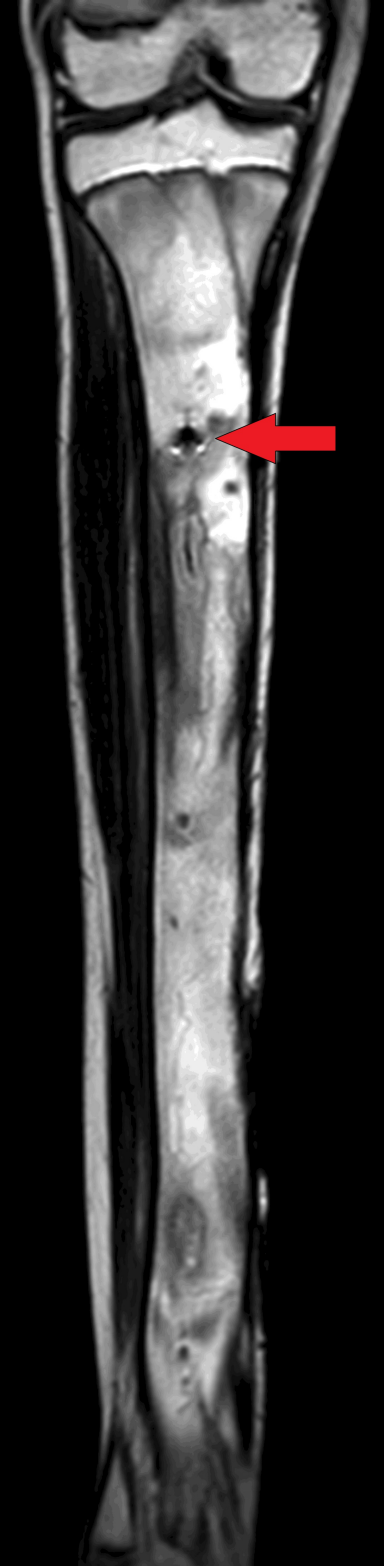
Coronal T1WI MRI of the tibia showing small areas of T1WI hypointensity, which do not show any enhancement in marrow suggestive of sequestrum. T1W1: T1-weighted imaging
